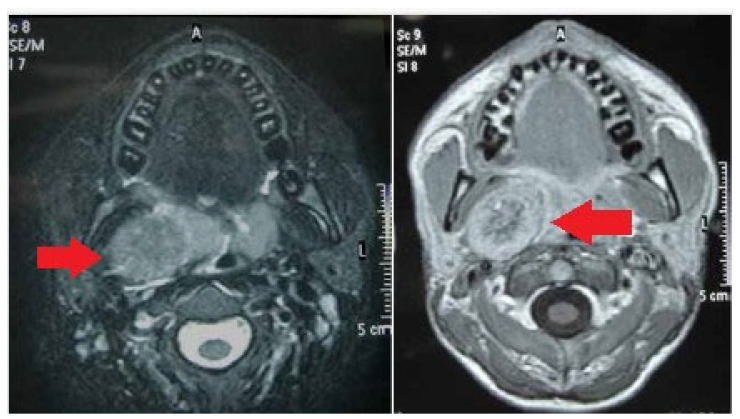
MRI imaging of a vagal schwannoma (red arrow) on the right cervical side.
